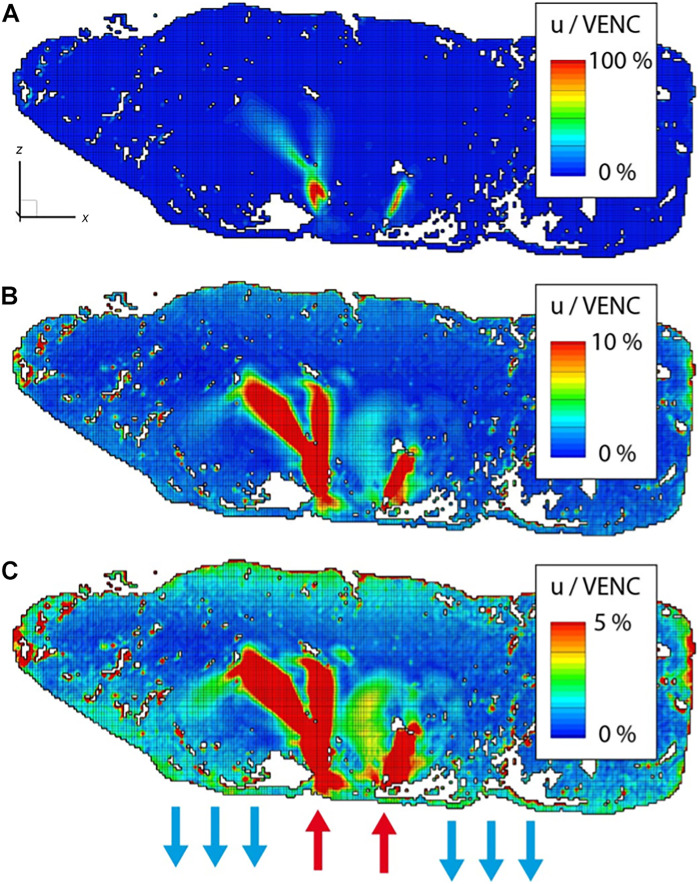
Contour plots of the VENC value. Dynamic range of the 3D MRI velocity measurement demonstrated at a characteristic position. Contour plots show the velocity values up to 100% of the VENC value (A), up to 10% of the VENC value (B), and up to 5% of the VENC value (C). Colored arrows indicated the number of inlets and outlets and their approximate positions.
